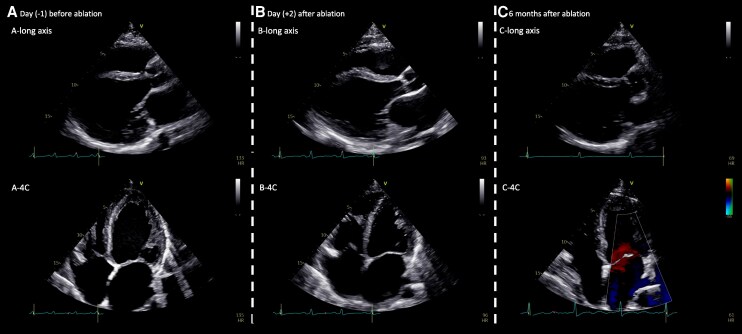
Reversal of left ventricular dysfunction following successful catheter ablation of permanent junctional reciprocating tachycardia–induced severe cardiomyopathy: echocardiographic images. (*A*) Parasternal long-axis and apical four-chamber views at presentation showing a markedly dilated left ventricle (LVEDD 63 mm) with severely reduced systolic function (LVEF 14%). (*B*) Immediate post-ablation echocardiogram demonstrating a reduction in left ventricular size (LVEDD 58 mm) and partial improvement in contractility (LVEF 26%). (*C*) Six-month follow-up echocardiogram showing near normalization of chamber dimensions (LVEDD 52 mm) and recovered systolic function (LVEF 50%).
